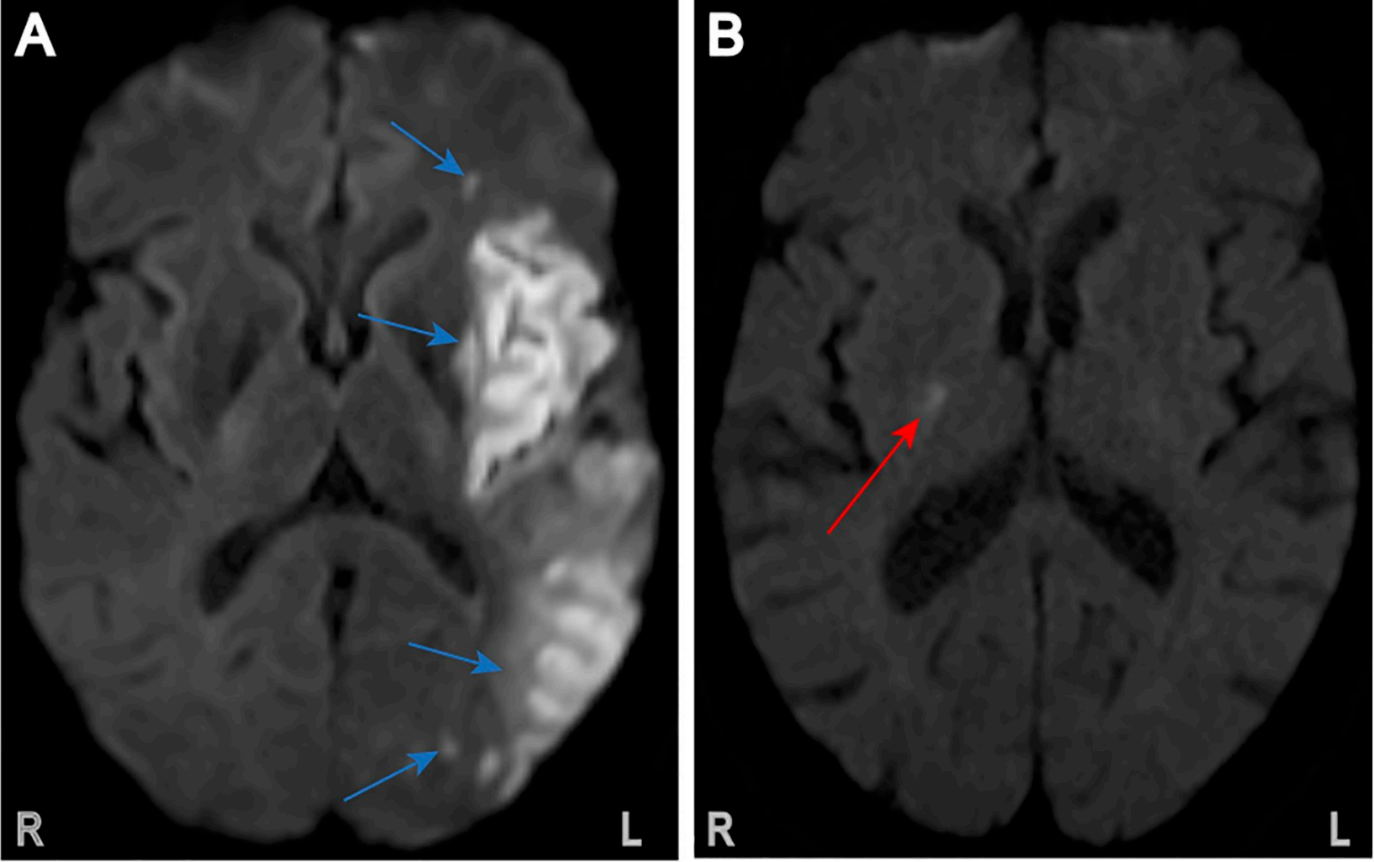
Sample patient brain magnetic resonance images demonstrating acute ischemic stroke patterns on T2 diffusion-weighted images. (A) Adjudicated cardioembolic stroke with multiple lesions (blue arrows) in left middle cerebral artery distribution. Total calculated infarct volume: 105.4mL. (B) Adjudicated small-vessel occlusion stroke with single lesion (red arrow) in the posterior limb of the right internal capsule. Total calculated infarct volume: 0.194mL.
